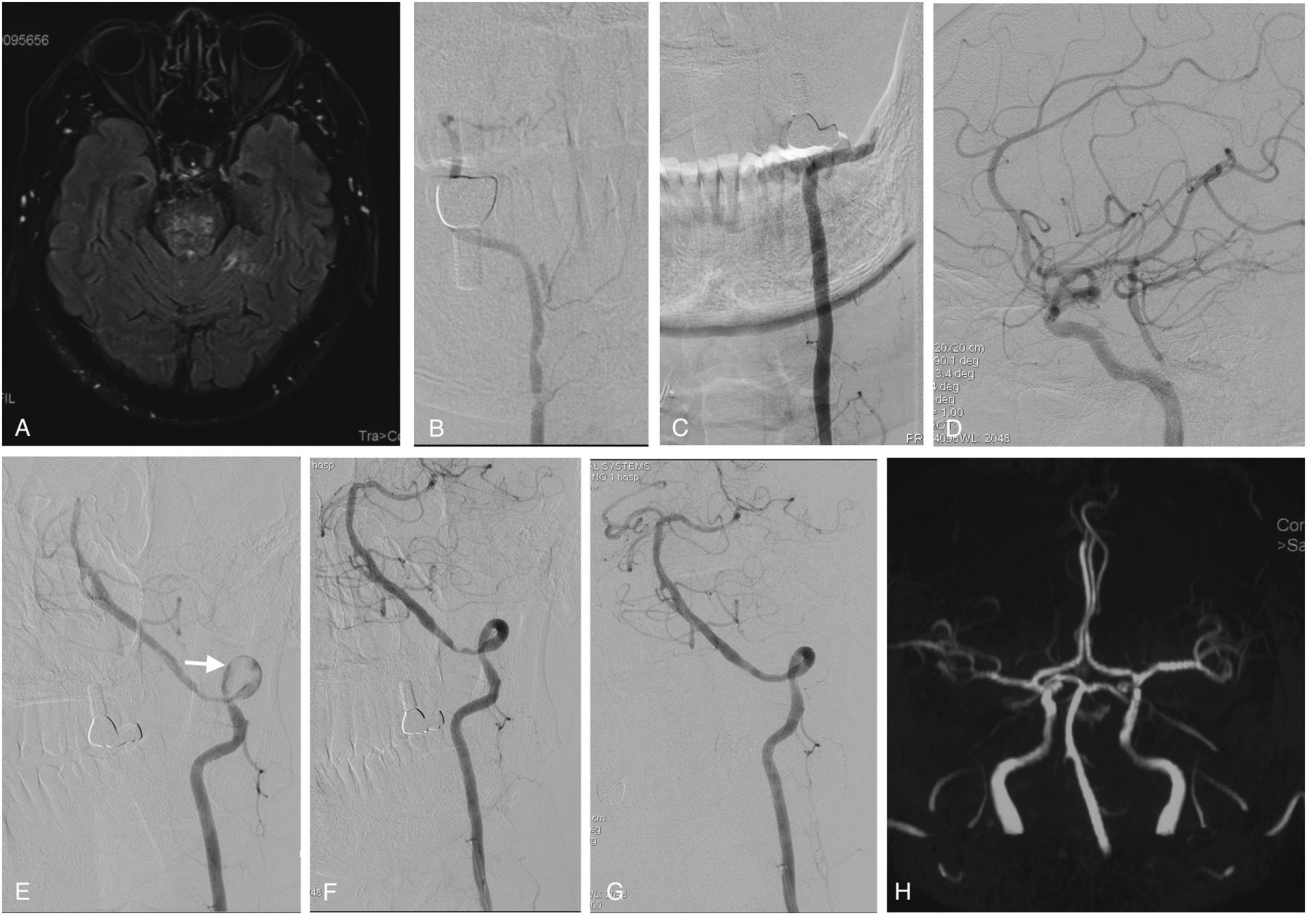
Imaging studies of case 1. Axial FLAIR MRI sequences showed ischemic infarctions in the pons (A) and bilateral cerebellum (not shown here). The right vertebral artery was hypoplastic (B), and the left vertebral artery was occluded from the V3 segment onward (C). The right PCOM supplied the PCA with retrograde flow to the top of the BA (D). In the first stage of endovascular treatment, the left intracranial vertebral artery was recanalized by passing a microwire through and dilating a balloon in the stenotic region; some thrombus material remained on the vessel wall (white arrow) (E). In the second stage of endovascular treatment, vertebral angiography showed that the thrombus had disappeared, leaving only the primary stenosis (F), which was fully resolved by angioplasty with stenting (G). Follow-up MRA performed 6 months later showed that the perfusion of the left intracranial vertebral artery and BA was unobstructed (H). MRI, magnetic resonance imaging; FLAIR, fluid-attenuated inversion recovery; PCOM, posterior communicating artery; PCA, posterior cerebral artery; BA, basilar artery; MRA, magnetic resonance angiography.
